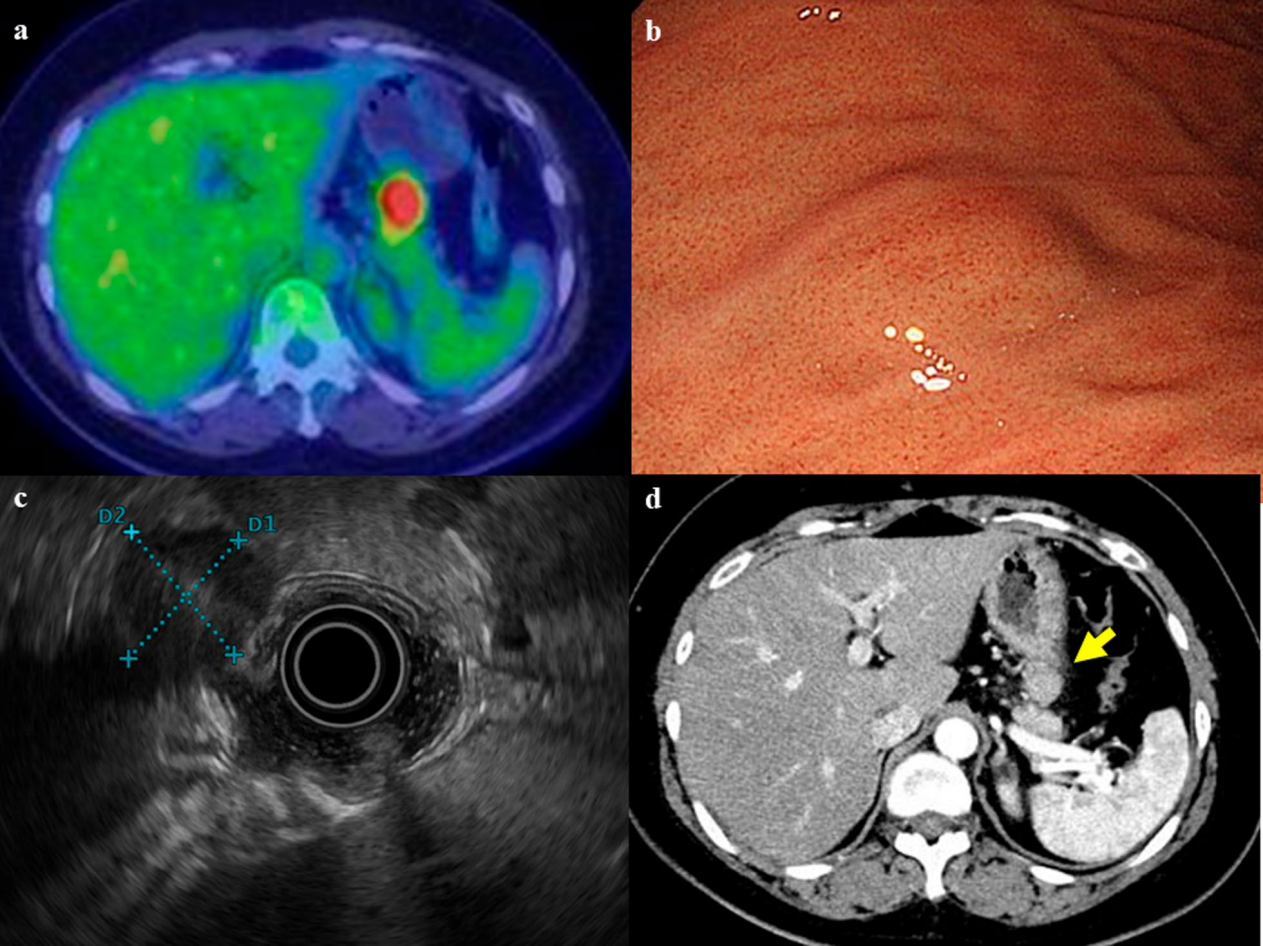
Positron emission tomography (PET) showed significant fluorodeoxyglucose accumulation (FDG) with a maximum standardized uptake value of 10.9 (a). Gastrointestinal endoscopic examination showed a smooth and protrusive lesion at the great curvature wall of the gastric body (b). Endoscopic ultrasound showed a hypoechoic exogenous mass and size of about 2.2 × 2.0 cm in the post wall of the stomach (c). Preoperative abdominal computed tomography scan showed an occupying lesion in the gastric wall (d). The arrow indicates the continuous mass on the stomach wall
